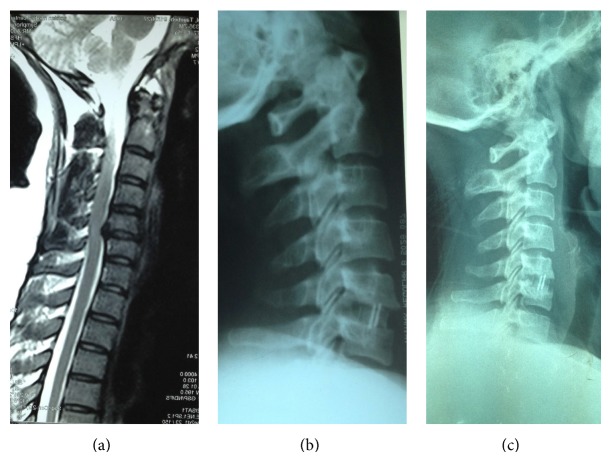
A 39-year-old female with C5-C6 cervical disc herniation (a). Lateral radiography on immediate postoperative day (b) was satisfactory but 26 months later frank pseudoarthrosis developed (c). The patient was completely asymptomatic.
